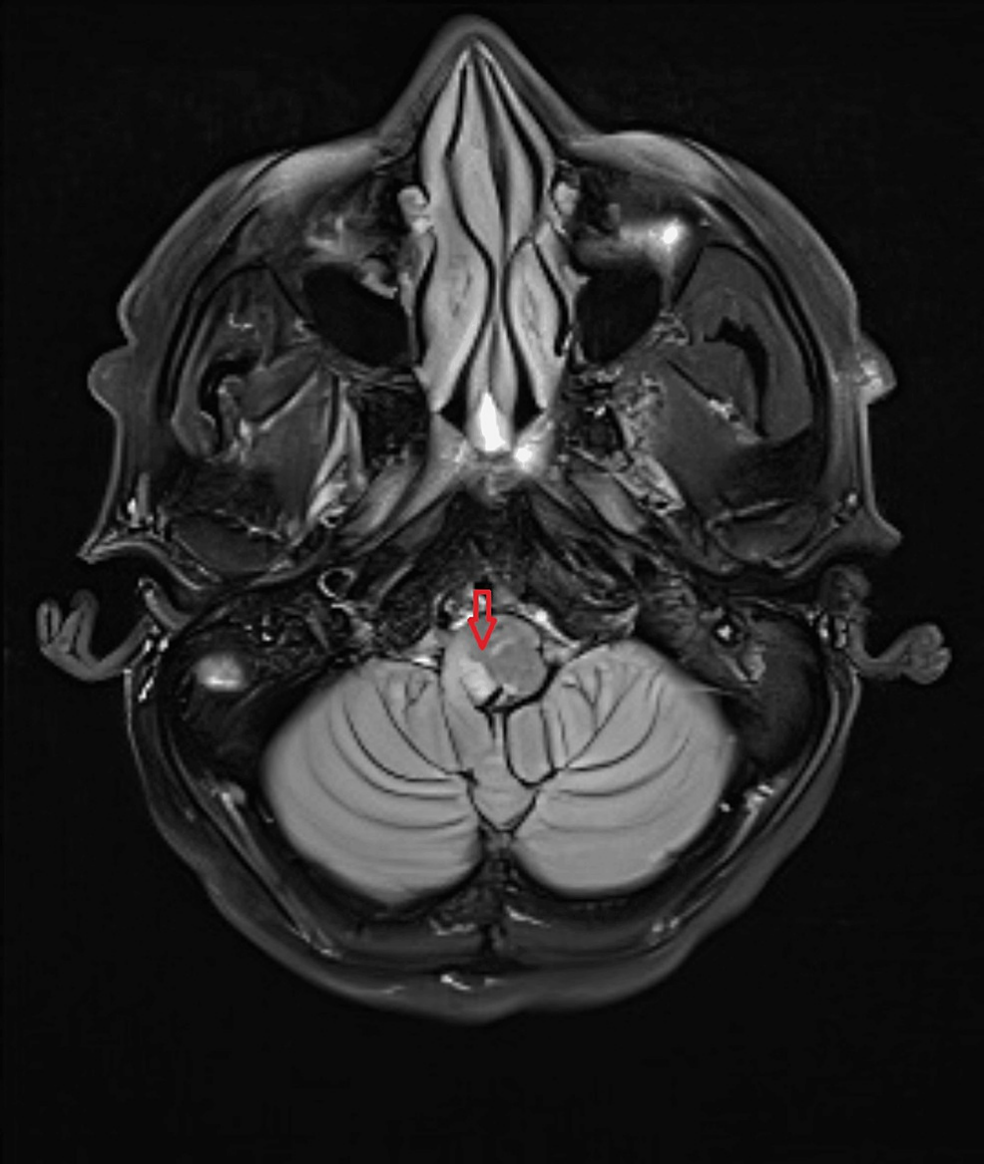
Axial brain MRI with posterior circulation infarct in the right medulla oblongata
